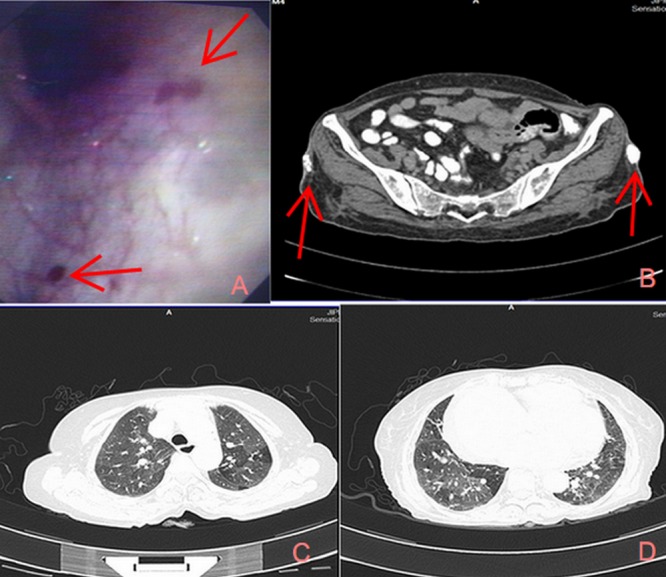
Investigations 2A: Telangiectasia in the gastric mucosa; 2B: CT abdomen revealed bilateral gluteal skin; 2C-2D: CT thorax - bilateral ground-glass appearance suggestive of interstitial lung disease
